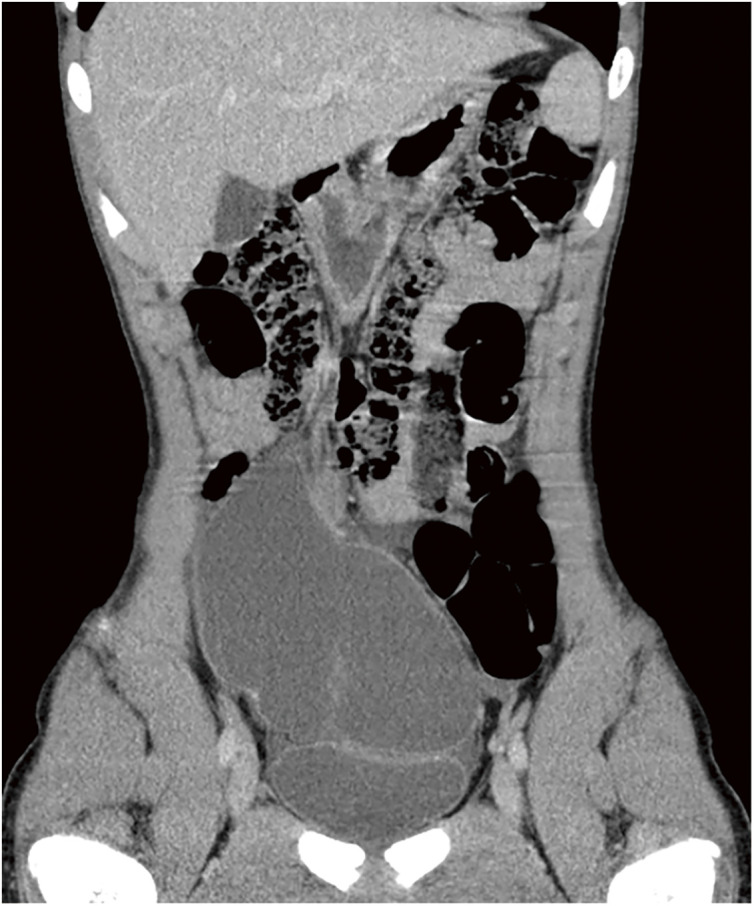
Computed tomography revealed a multicystic lesion in the right ileocecal region and ascites fluid in the lateral paracolic gutter.
